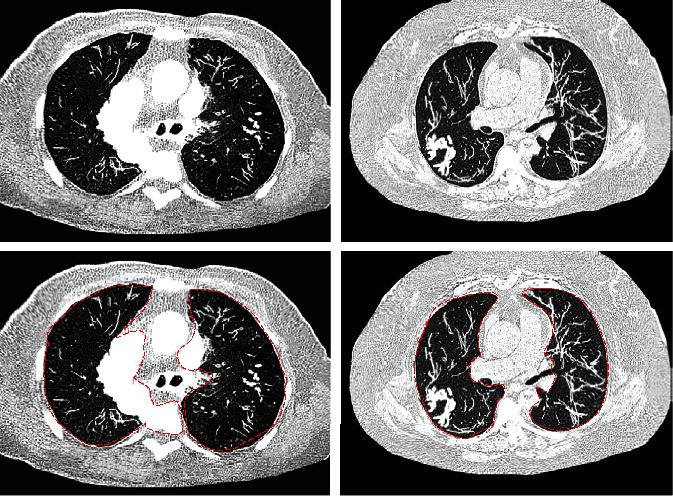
Comparison of the segmentation effect of single threshold segmentation algorithm on thoracic CT images. The area inside the red box was the feature extraction range; the dotted line was the feature contour extracted by the algorithm.
